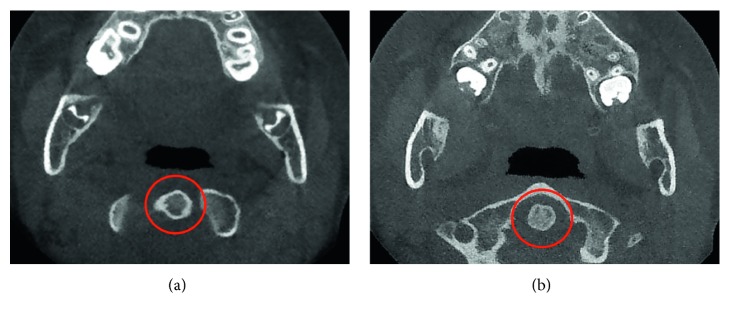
Transversal slice CT taken in closed mouth (a) and in maximum oral aperture (b). The circles represent the second cervical vertebrae's body, in the centered position.
